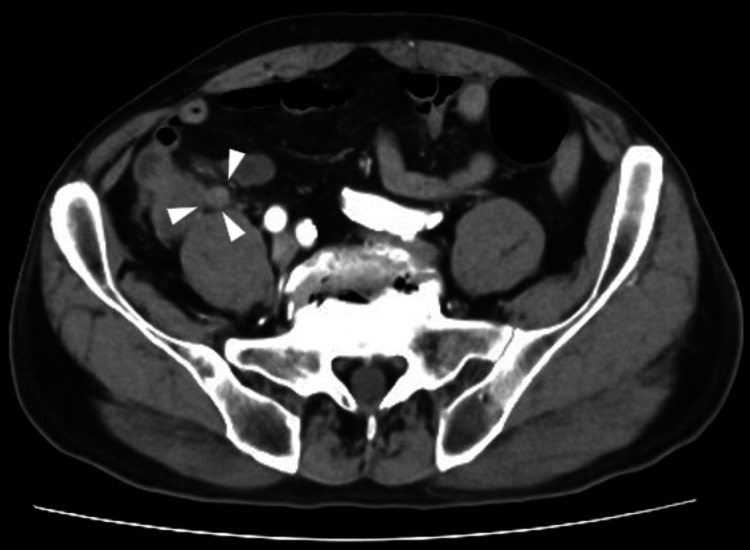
Abdominal CT The CT scan revealed inflammatory changes in the appendix (arrow).
